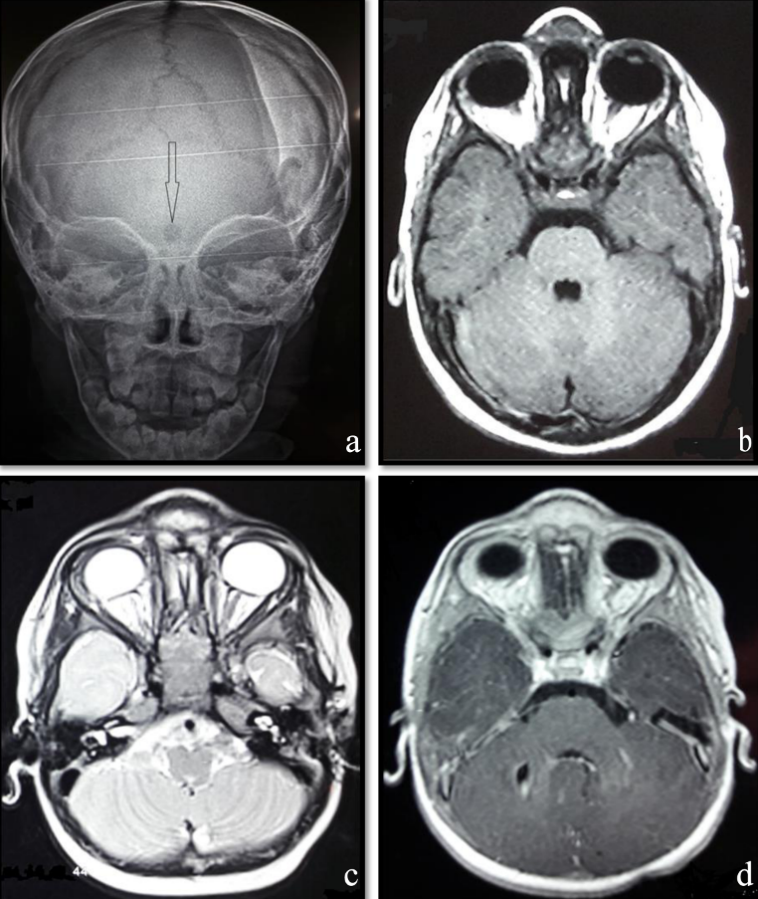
Frontal radiograph of the skull (a) showing nasofrontal bone defect and axial brain MRI weighted sequences T1 (b), T2 (c), and T1 contrast enhanced (d). The lesion sits in the medial basifrontal and appears hypointense on T1 and hyperintense on T2 sequences, and enhances after injection of the contrast product (d).
